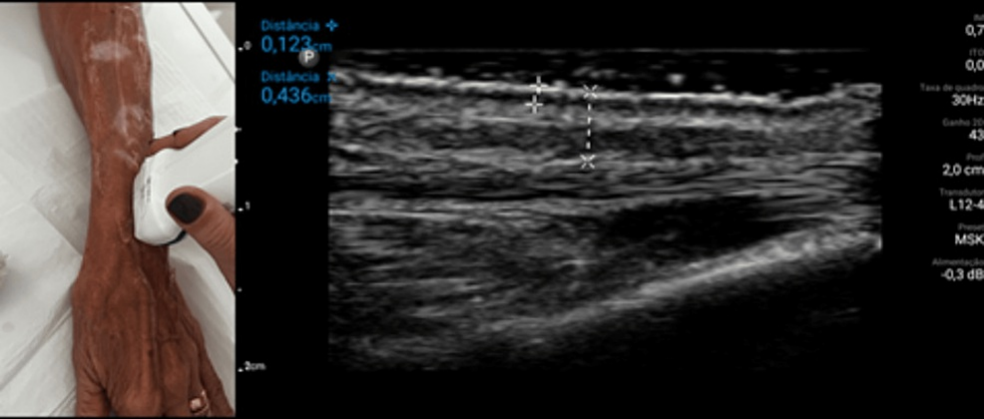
Ultrasound at nine months.
